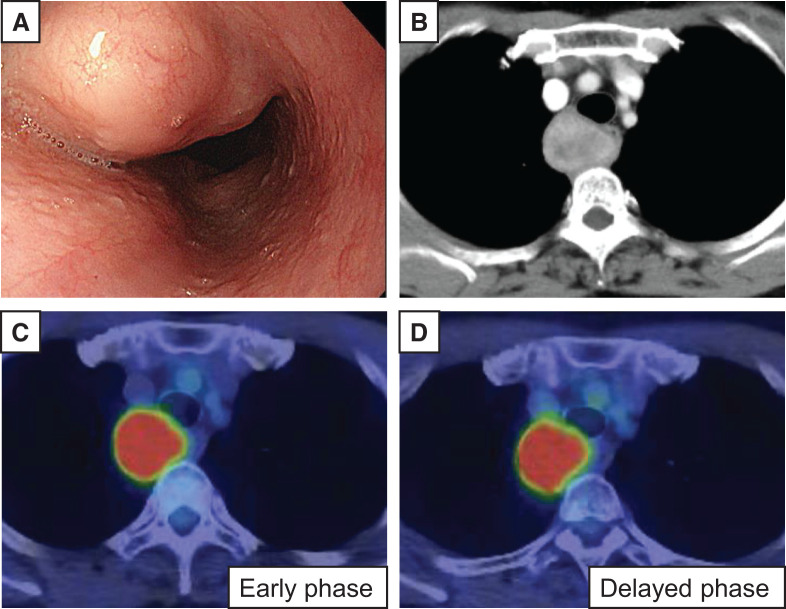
(A) Upper gastrointestinal endoscopy revealed a well-demarcated, smooth-surfaced lesion in the upper esophagus, 17 cm from the incisors, without luminal obstruction. (B) Chest CT identified a 34 × 27 mm mass on the right side of the upper thoracic esophagus, exerting compression on both the trachea and esophagus. (C, D) FDG-PET revealed elevated uptake, with an early SUVmax of 9.4 (C) and a delayed SUVmax of 11.8 (D). FDG-PET, fluorodeoxyglucose positron emission tomography; SUVmax, maximum standardized uptake value
